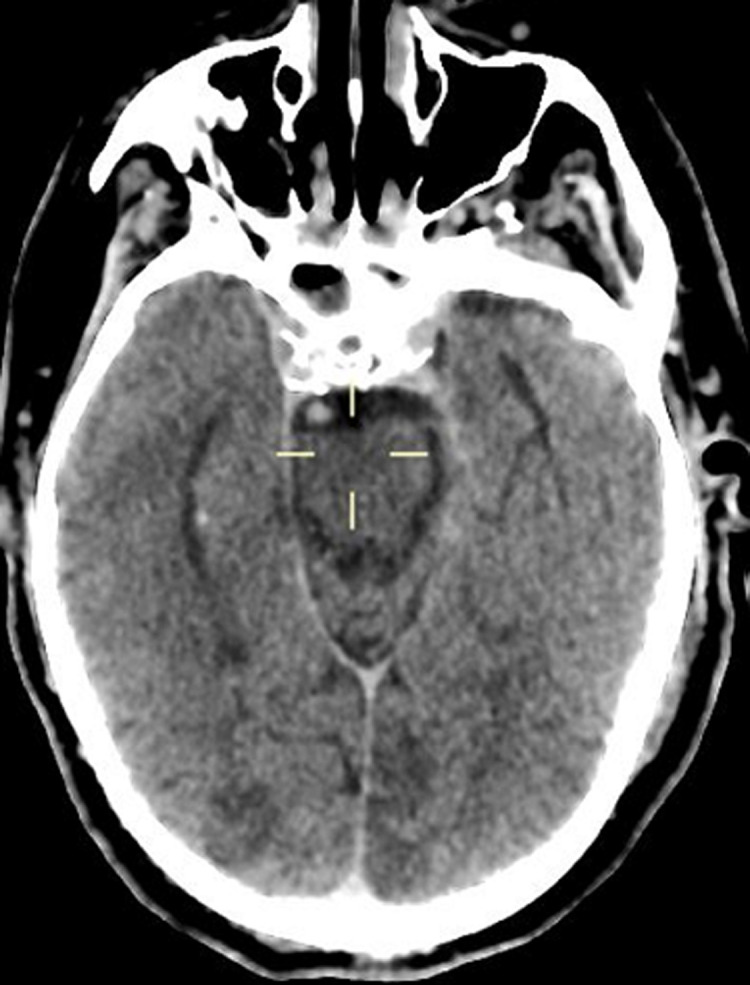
cerebral CT scan revealing multiple hypodense areas cortico-subcortical bilateral parietal and occipital related to multiple strokes
